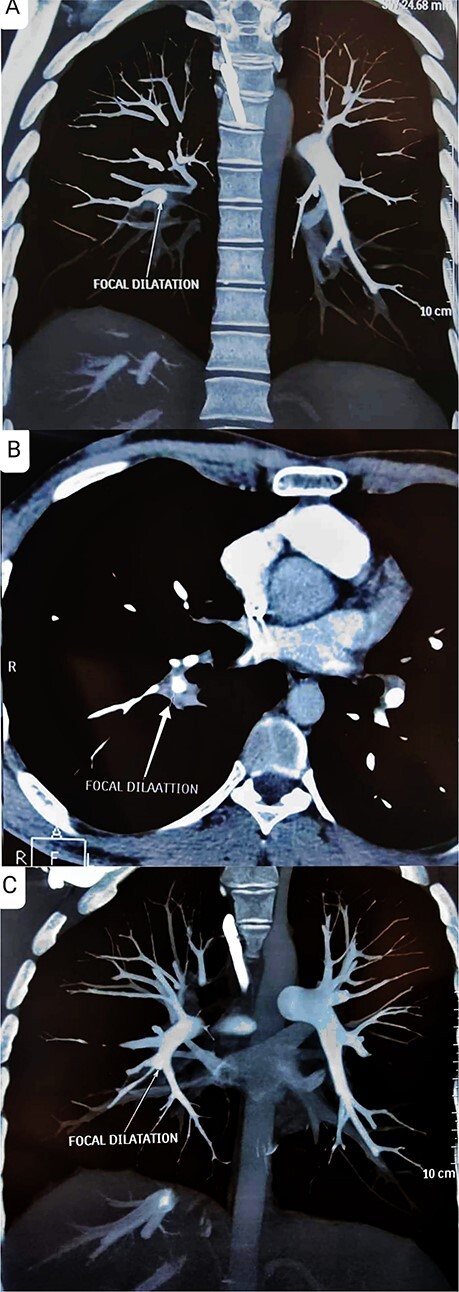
(A–C) CT pulmonary angiogram shows focal dilation of approximately 8 mm observed at the right lower lobe pulmonary artery, precisely at the first-order bifurcation (white arrows) and no fibrosis or parenchymal lung changes were detected.
